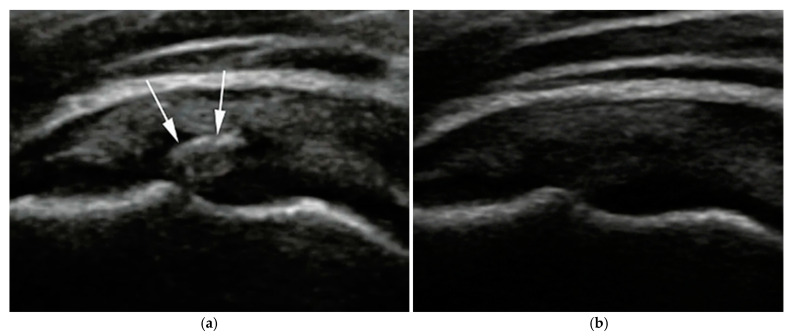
Representative ultrasound images of calcific deposits in a patient treated with ultrasound-guided ESWT. (a) Baseline image showing a well-defined calcific deposit (white arrows); (b) Six-month follow-up demonstrating complete resorption, with no visible echogenic material.
